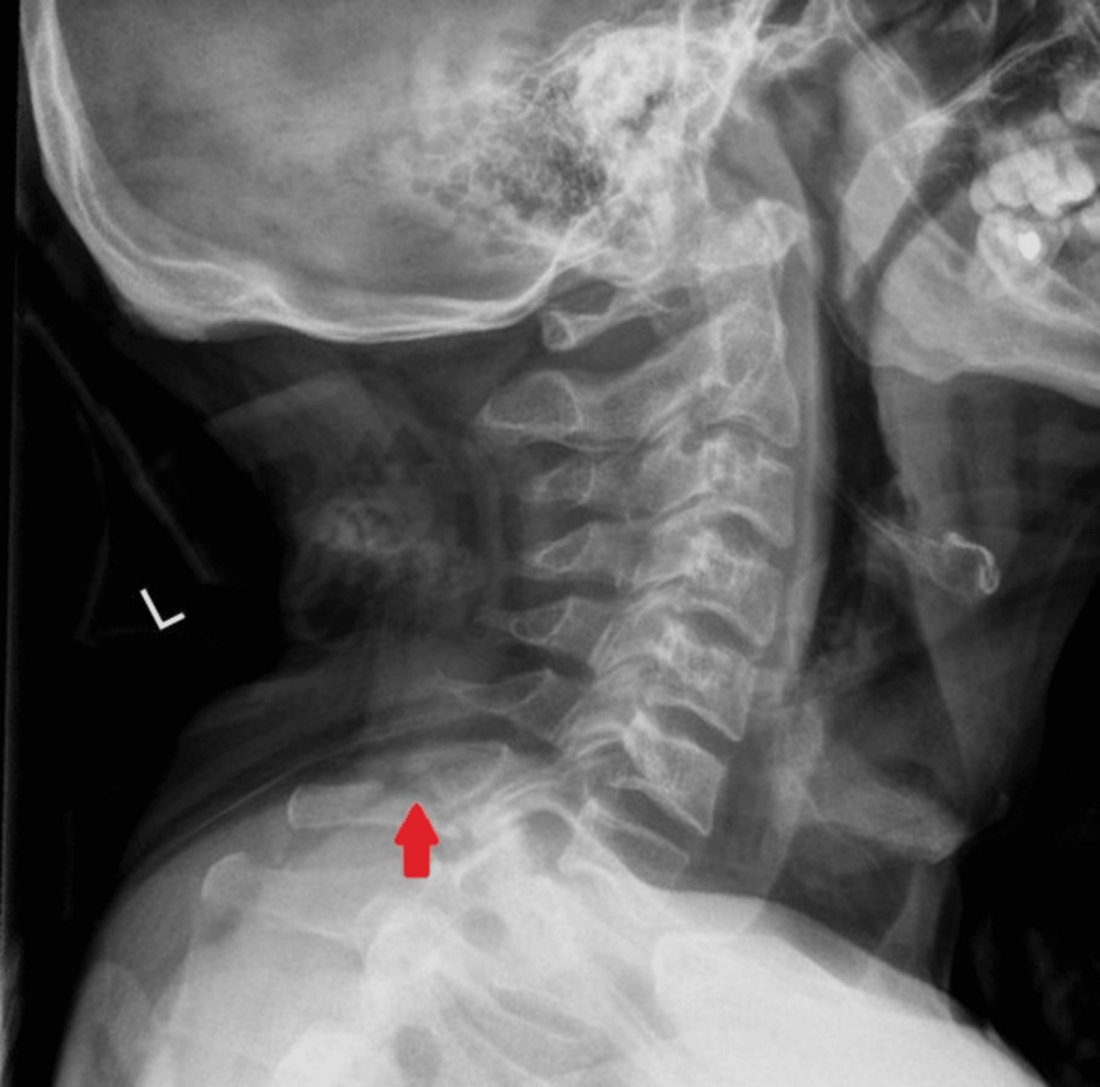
Lateral view cervical spine X-ray showing the previously identified C7 spinous process fracture (red arrow).
